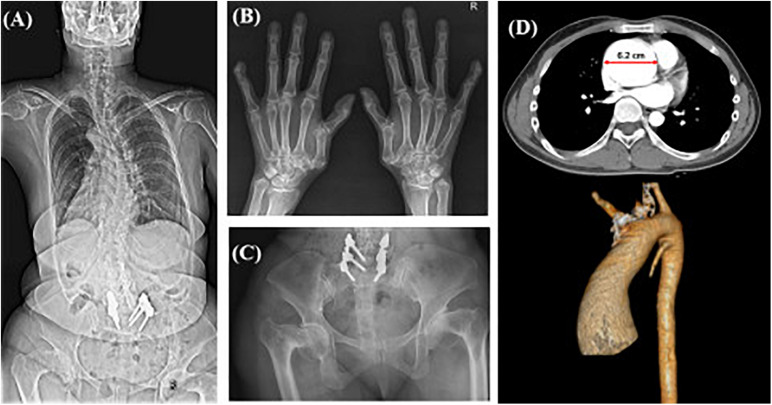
Radiographic images of case #13 and affected mother with aneurysms-osteoarthritis syndrome. X-ray images of the patient’s affected mother showing (A) severe scoliosis despite surgical correction, (B) degenerative osteoarthritis of the hand joints with subluxation of the 1st carpometacarpal joints, (C) bilateral severe coxa vara deformities; (D) CT scan with 3D reconstruction of Case #13 showing aneurysm of the ascending aorta with a dilated root (diameter: 6.2 cm).
